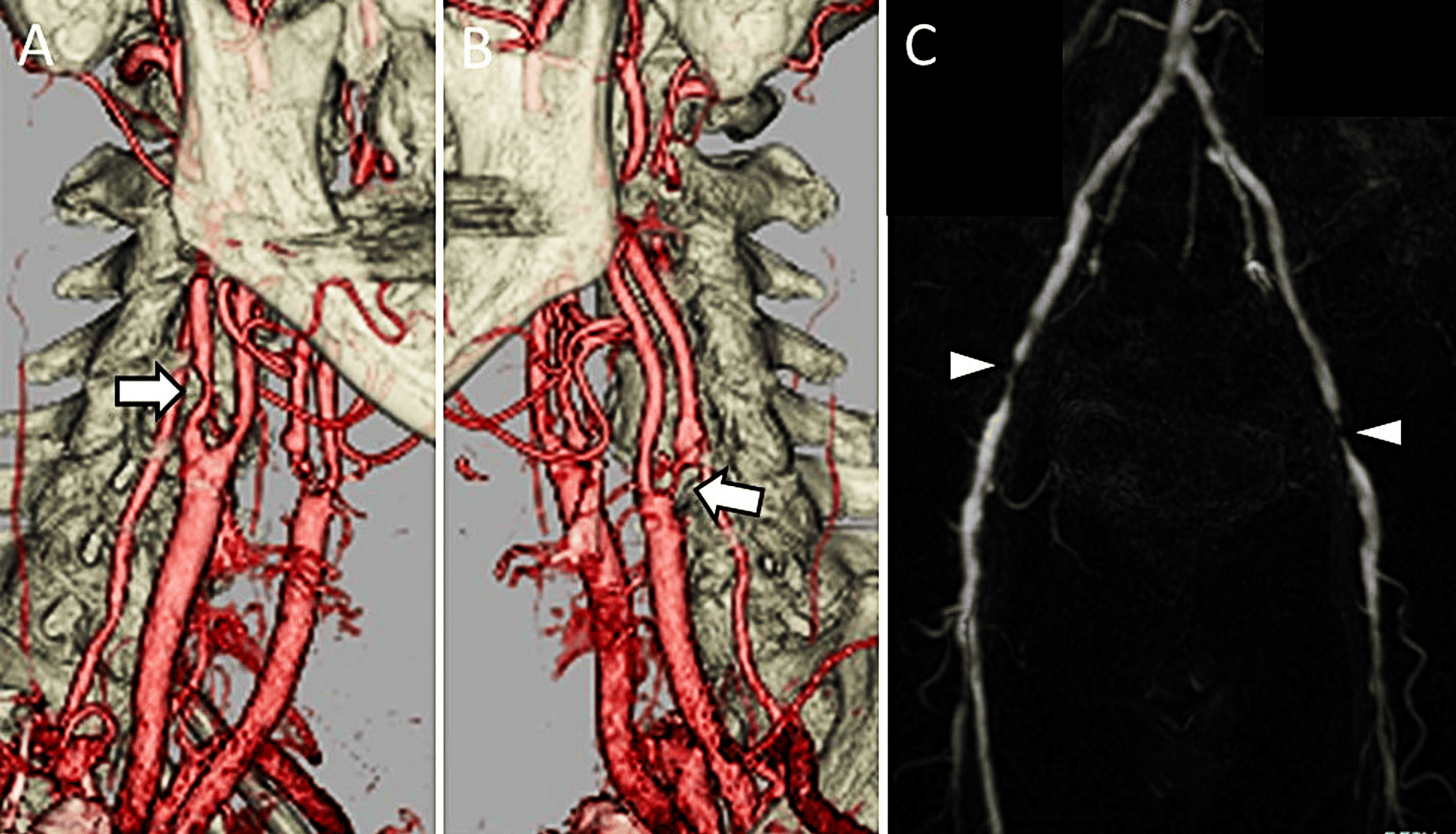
Preoperative images. (A,B) Cervical three-dimensional computed tomography angiography shows bilateral internal carotid artery stenosis (arrows). (C) Lower extremity magnetic resonance angiography demonstrates bilateral external iliac artery stenosis (arrowheads)
